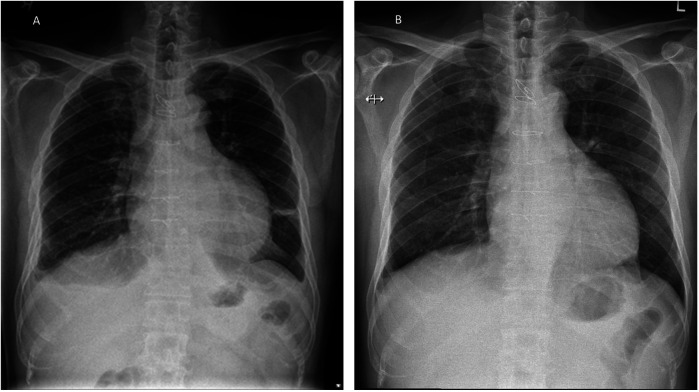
Patient's chest x-ray. (A) CXR during his admission for pericardial tamponade showing a globular heart and small pleural effusion. (B) Outpatient CXR taken 8 days later, showing that the third and fourth sternal wires had fractured with widening of the fifth and sixth wires.
